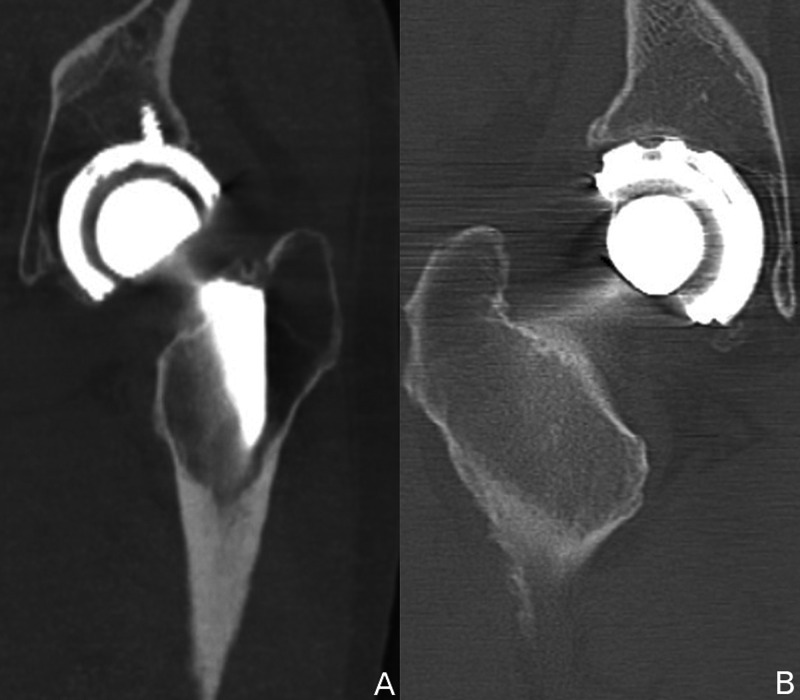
Excessive cup lateralization contributing to IPI. (A) Coronal CT scan of the left hip showing cup placement lateral to the base of the fossa. (B) Coronal CT scan of the right hip showing vertical cup and lateral placement relative to the fossa. IPI, iliopsoas impingement; CT, computed tomography
